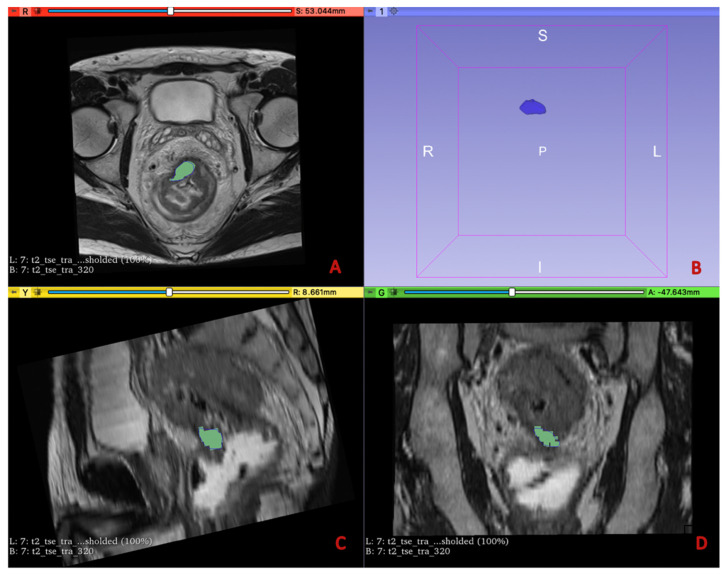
The image-processing software 3D Slicer was used to manually outline ROIs along the lesion margins on all the slices containing the tumor in the MRI T2w sequences (A,C,D) and ADC sequences, as shown in the figure. The volumetric 3D tumor reconstruction is shown in the upper right quadrant (B).
